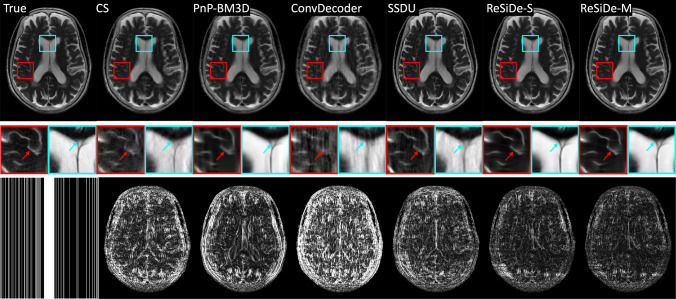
An example showing reconstruction of a T2-weighted image with sampling mask M2. To highlight differences, the second row magnifies two areas in the brain. The arrows point to features where some of the methods show artifacts or blurring. The third row shows the sampling mask (left) and the absolute error maps after five-fold amplification
